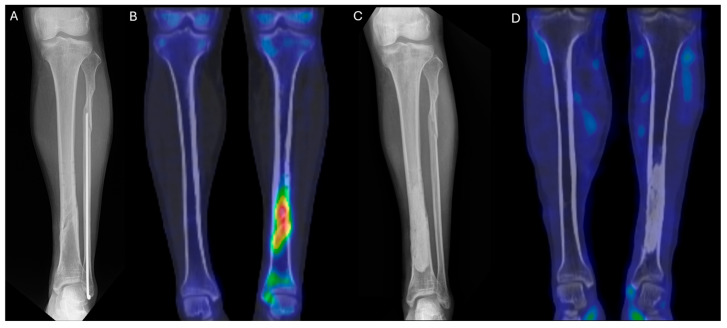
(A) Preoperative lower leg AP X-ray. (B) Preoperative ^99m^Tc DPD bone SPECT/CT coronal image. (C) Postoperative lower leg AP X-ray at 1 month. (D) Postoperative ^99m^Tc DPD bone SPECT/CT coronal image at 6 months, showing no hypermetabolic uptake, consistent with infection control.
